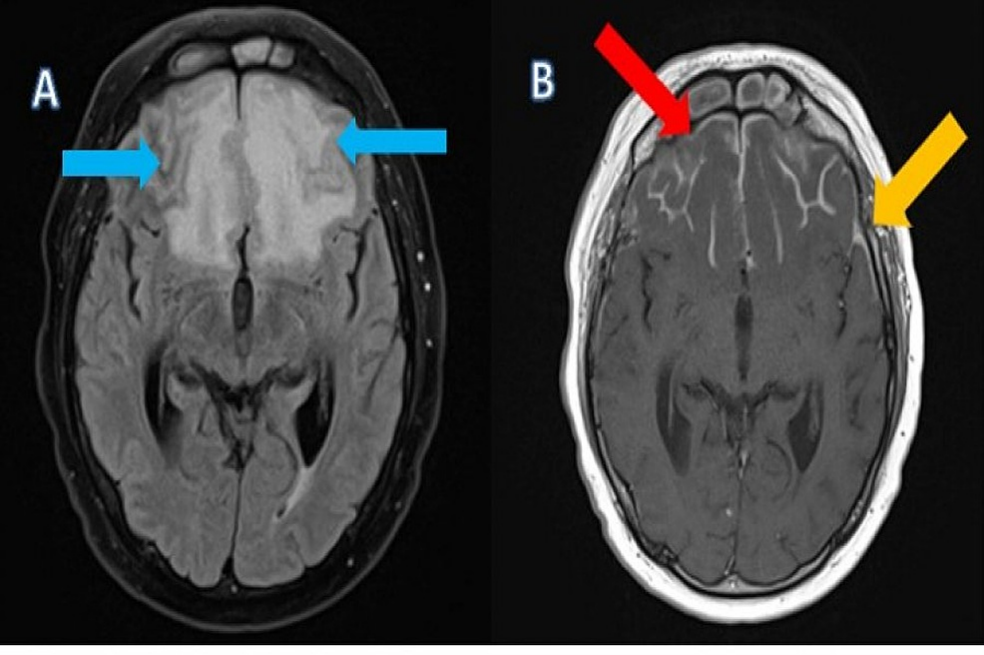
(A) MRI, axial T2 FLAIR demonstrating extensive oedema in the frontal lobes bilaterally (blue arrows). (B) MRI, axial T1 post-contrast, demonstrating leptomeningeal enhancement overlying the frontal lobe gyri (red arrow) and extending to the temporal lobes (orange arrow).
